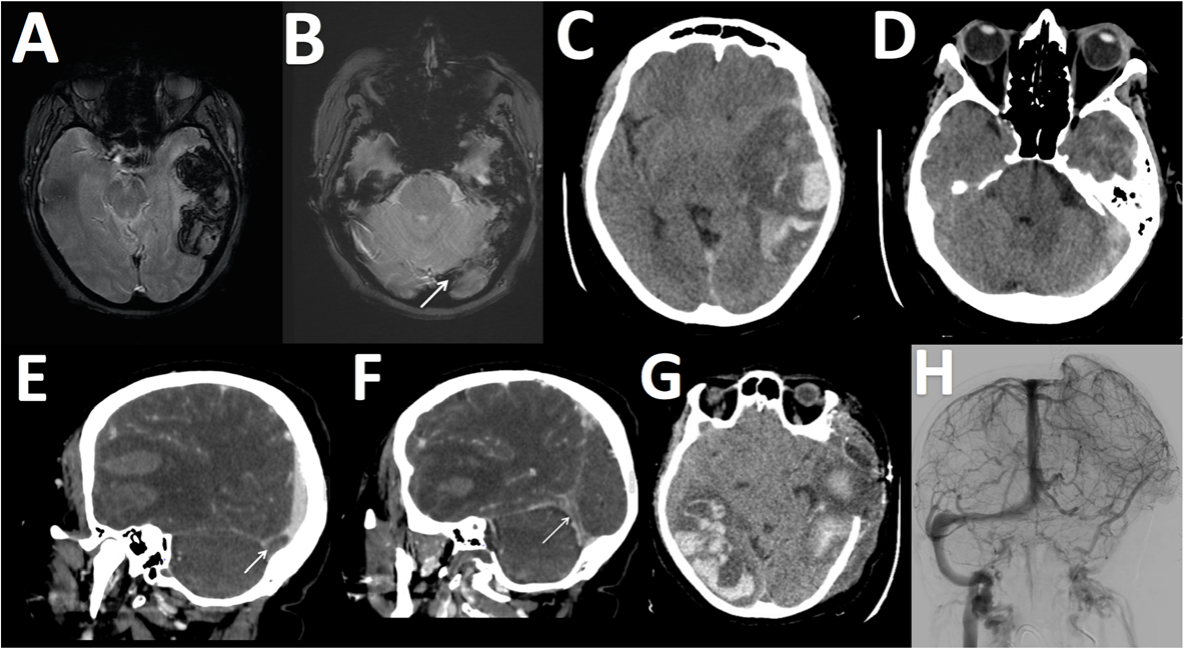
MRI, venous CT scanner, and cerebral angiography at admission and day 14. MRI images (a, b) demonstrates voluminous left temporal hemorrhage with venous thrombosis (arrow). Venous CT scanner (c–f) confirms the existence of the extensive venous thrombosis. Located in the straight sinus and left lateral sinus (arrow). Day 14 CT scanner shows contro-lateral. Brain hemorrhage (g) and cerebral angiography shows persistent left thrombosis (h)
